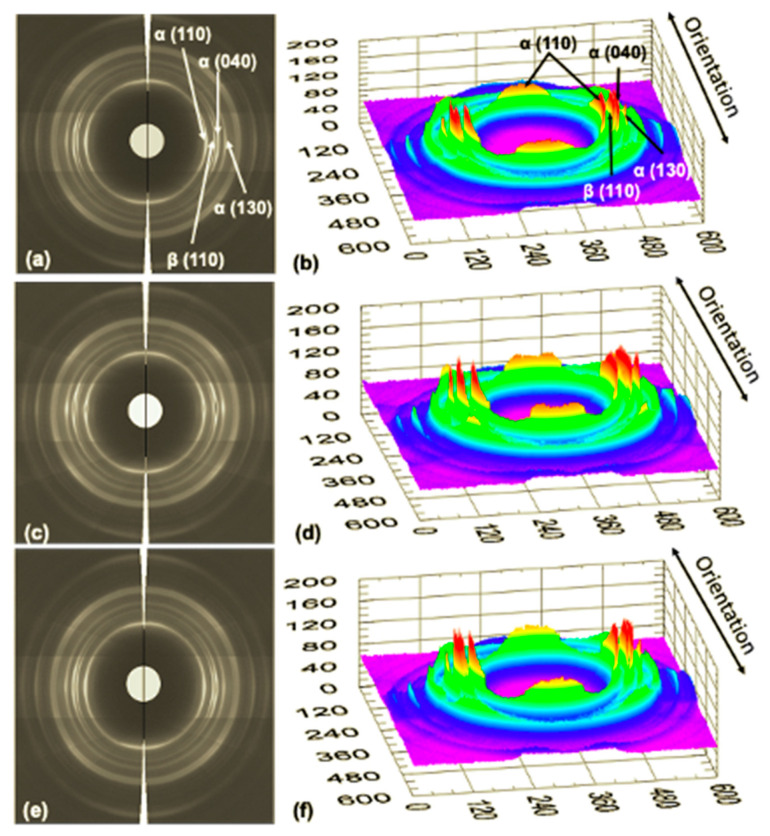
2D and 3D WAXS patterns of the iPP probes injected at constant ultrasound time (6 s) and different amplitudes: (a,b) 80%, (c,d) 90%, and (e,f) 100%. The primary crystalline reflections of the α crystalline phase: (110), (040), and (130), and β crystalline phase (110) of iPP are also shown.
